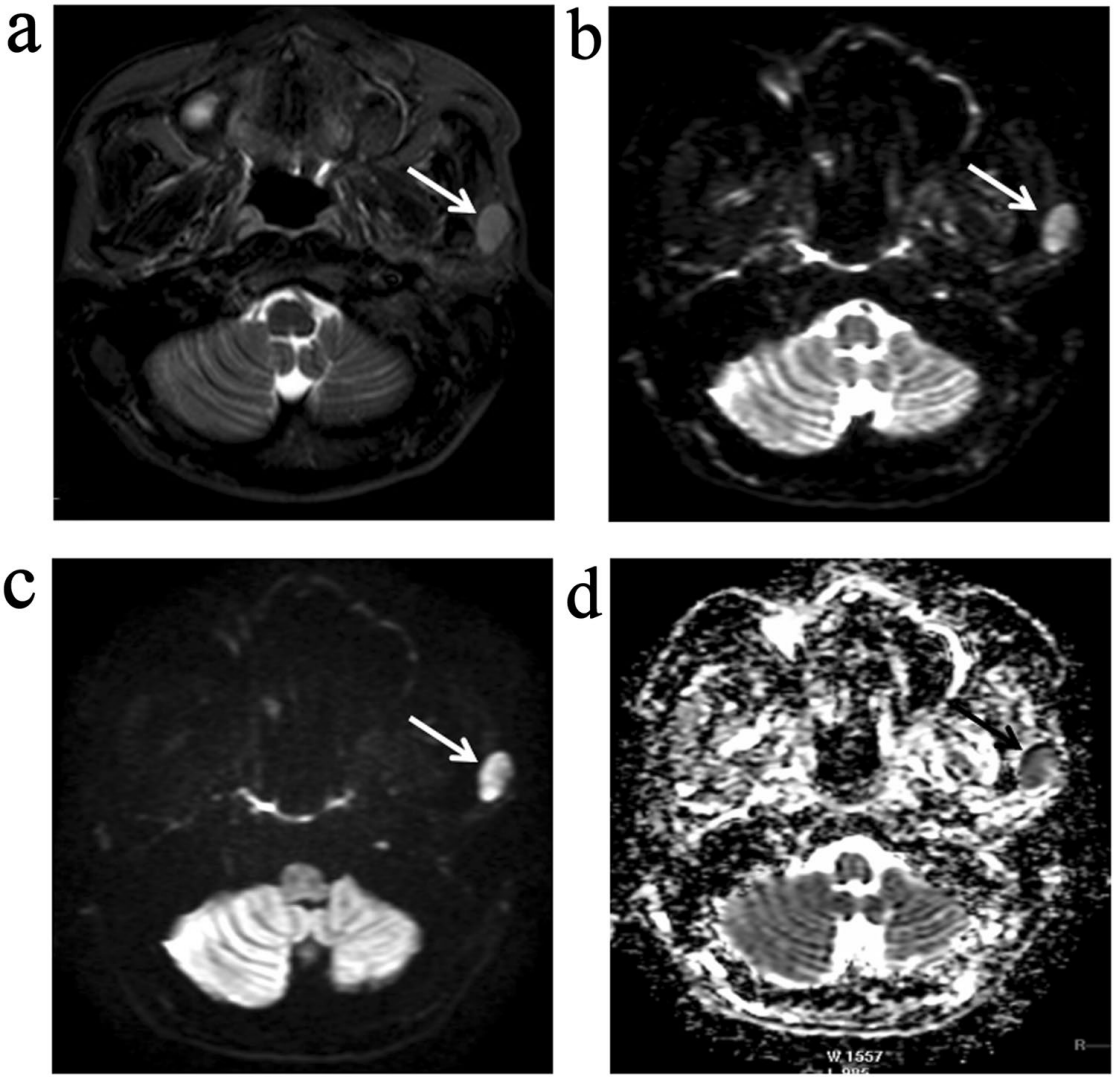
MR images of a metastatic PLN which includes both necrotic and solid portions (arrows) on a transverse T2-weighted image (a). Axial diffusion-weighted MR images show heterogeneous signal intensity, including hypointensity of necrotic part at *b* = 0 s/mm^2^ (b) and decreased signal intensities at *b* = 800 s/mm^2^ (c) compared to the solid components of the malignant PLN. In contrast with solid portions, the necrotic areas show hyperintensity on the ADC map (d).
